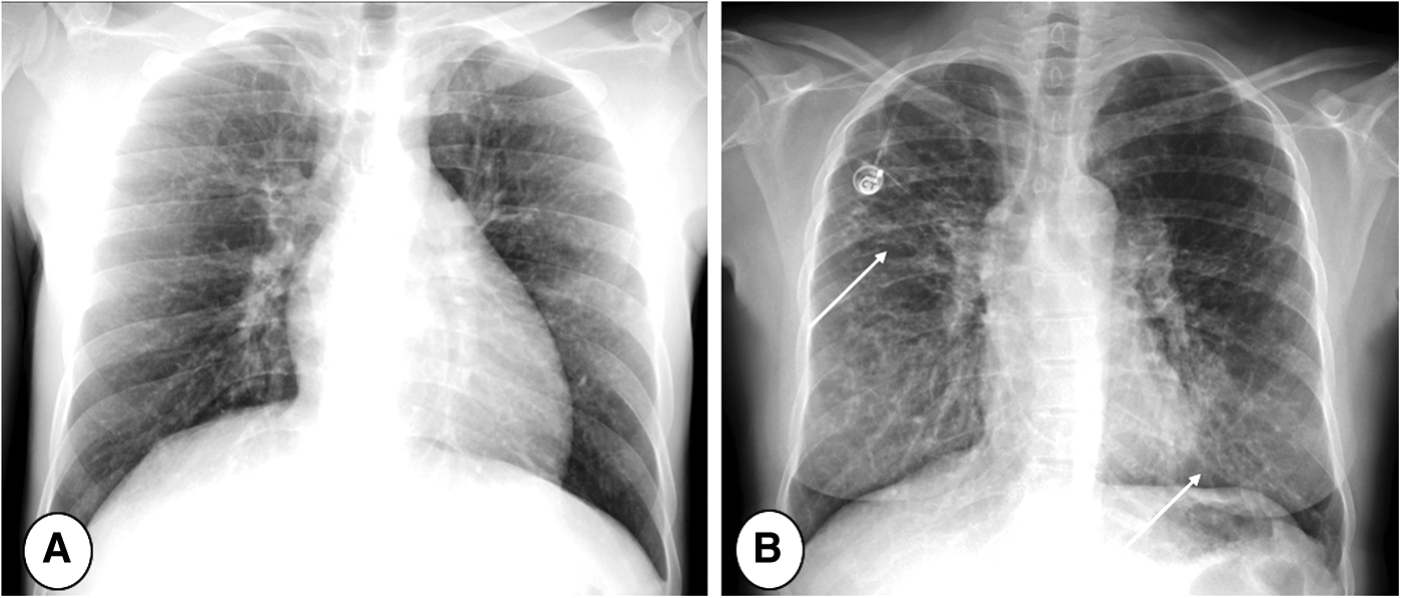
Posterior/anterior chest radiographs in (A) mild and (B) severe cystic fibrosis lung disease. Note the progressive increase of bronchial pathology in the subject with severe disease (B, arrows). The more severe the disease, the more difficult the detection of new abnormalities, especially during respiratory tract exacerbation.
